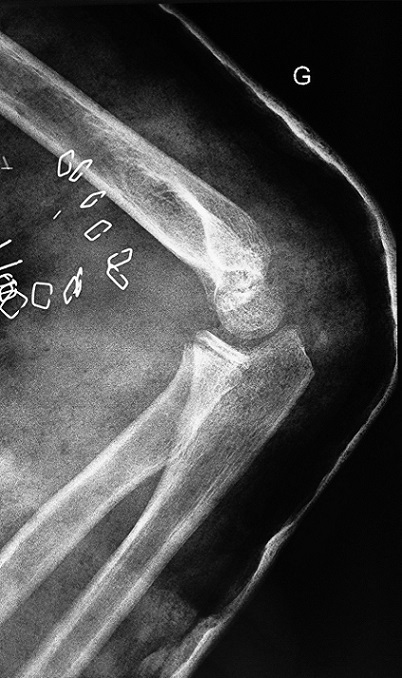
Elbow radiograph after reduction (profile)
